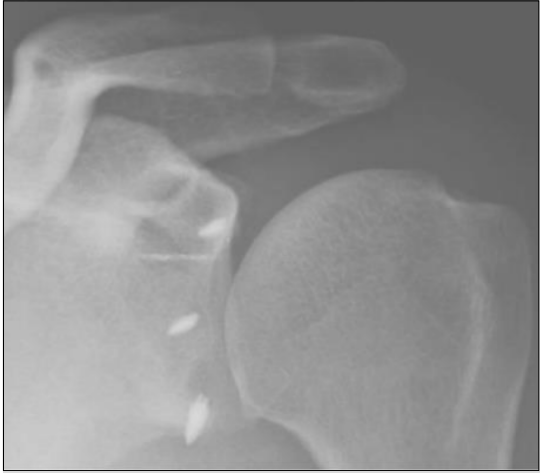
True anteroposterior radiograph showing anchor positioning.
